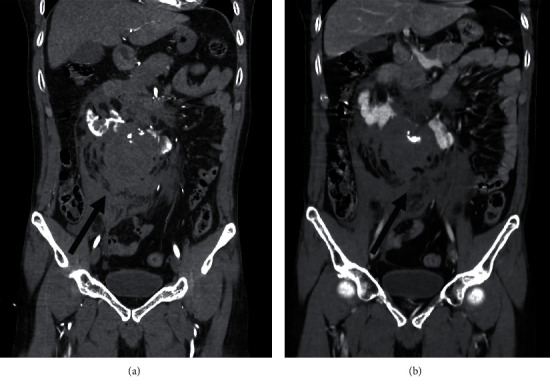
CT angiography in coronal view, showing an active contrast extravasation from accessory right renal artery and retroperitoneal hematoma (a, b) (black arrow).
